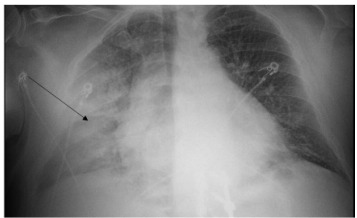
Initial emergency department chest radiograph with evidence of acute right-sided “flash” pulmonary edema (arrow).
